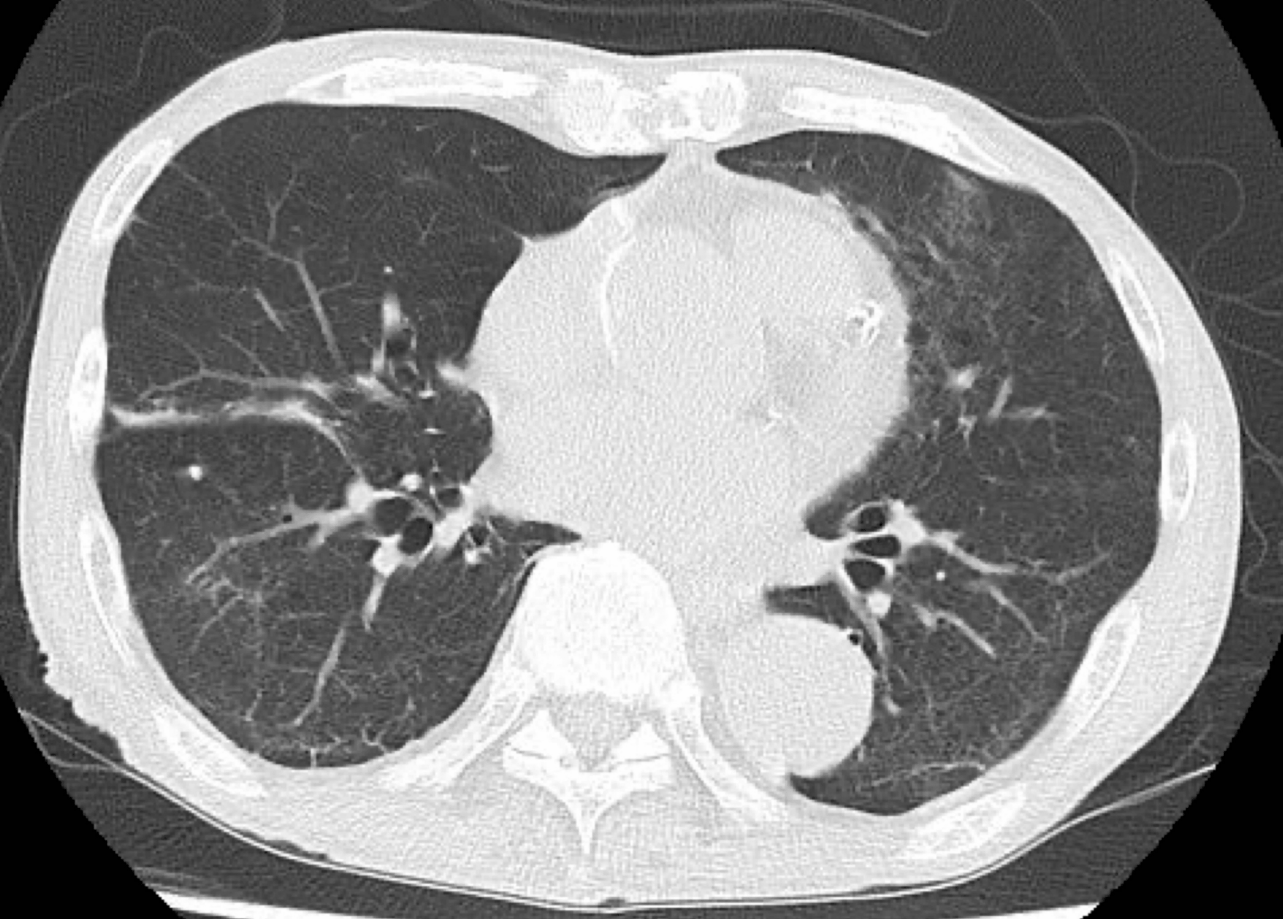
Follow-up chest CT on hospital day 27 Chest CT showing resolution of bilateral ground-glass opacities following completion of three weeks of treatment with trimethoprim-sulfamethoxazole and atovaquone.
